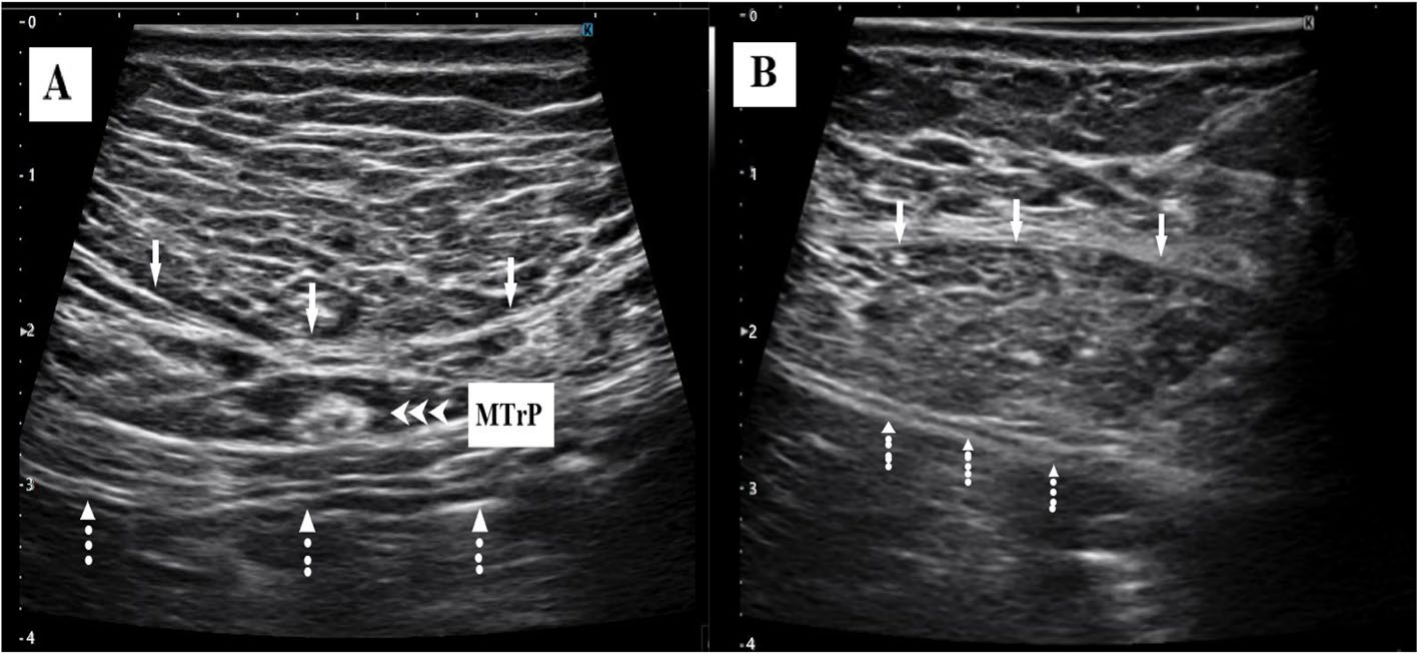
Ultrasound image of the internal oblique abdominal muscle at the second (A) and the fifth visit (B). A spherical hyperechoic spot with a major axis diameter of 5 mm surrounded by a hypoechoic area is shown at the myofascial trigger point in the internal oblique abdominal muscle (A). The hyper- and hypoechoic area disappeared, which changed to an irregular muscle bundle alignment at the same site of A and its vicinity (B). Vertical arrows indicate the outer fascia of the internal oblique abdominal muscle, and dotted arrows indicate the peritoneum. MTrP, myofascial trigger point
